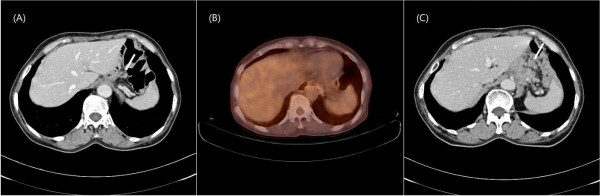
Contrast CT and PET/CT with lymph node recurrence. (A) Prominent lymph node in contrast CT suggesting recurrence (arrow) (B) PET/CT finding without hypermetabolic lesions suggesting absence of tumor recurrence (C) Increased previous lesion (arrow) after 6 months.
